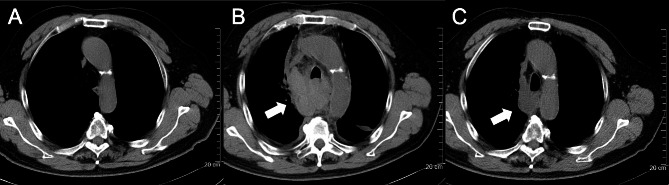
Chest computed tomography. (A) The pre-DSA image shows the normal mediastinum, trachea, and lung range. (B) The post-DSA image shows mediastinal hematoma and tracheal compression. (C) The image at discharge (after 48 days) shows decreased mediastinal hematoma and increased tracheal diameter
